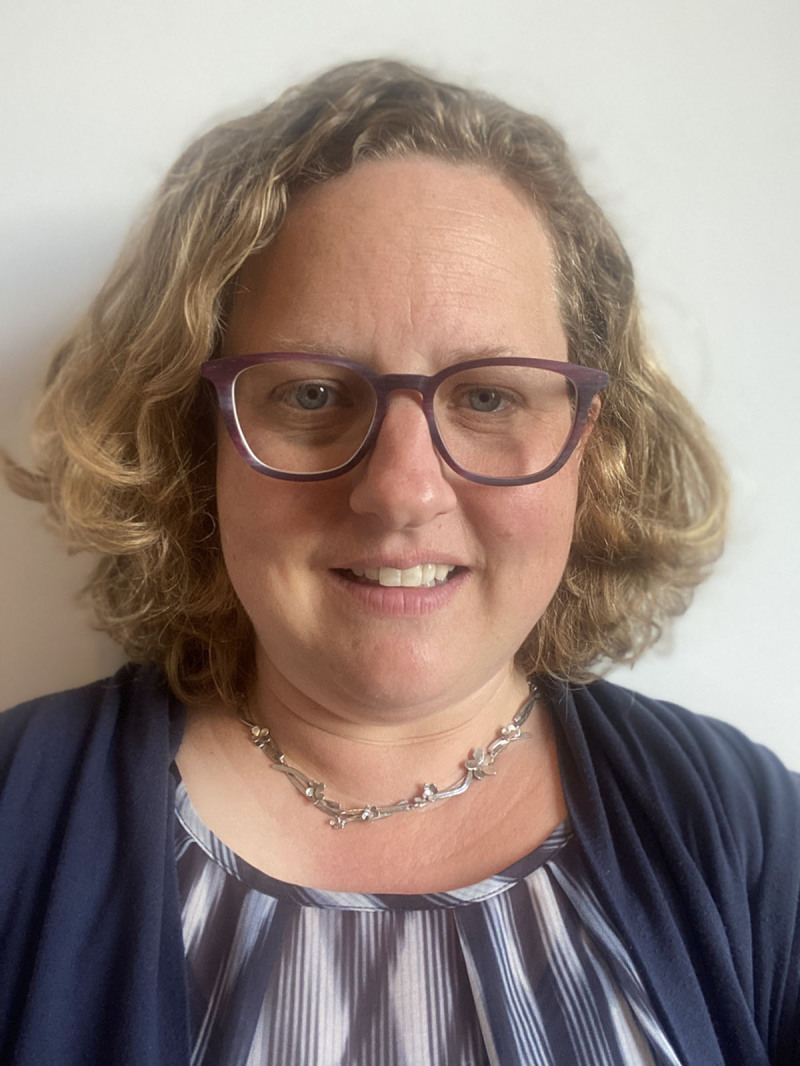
Dr. Annemiek Snoeckx (MD, PhD) is a radiologist at the University Hospital of Antwerp, Belgium. She graduated as Medical Doctor in 2003 at the University of Antwerp. She is a board-certified radiologist since 2008 and has been working since then at the University Hospital of Antwerp. Her main field of interest is chest imaging, with a focus on thoracic oncology imaging in general, pulmonary nodules, and lung cancer screening. She earned a PhD in 2019. She is an active member of the Belgian Society of Radiology (BSR), the European Society of Thoracic Imaging (ESTI), the European Society of Radiology (ESR), the European Respiratory Society (ERS) and the International Association for the Study of Lung Cancer (IASLC). She is the Dutch-speaking representative of the BSR chest section. On a European level, she is a member of the ESTI Training and Educational Committee, Diploma Committee and Lung Cancer Screening Certification Project. She is team leader of the Chest Radiology Written Evaluation Committee for the European Diploma in Radiology (EDIR). Her research interests are in the field of pulmonary nodules, artificial intelligence, lung cancer screening and Dual-Energy CT in lung cancer imaging. She authored more than 60 papers in peer-reviewed journals and eight book chapters. She has lectured at many national and international meetings and courses.
